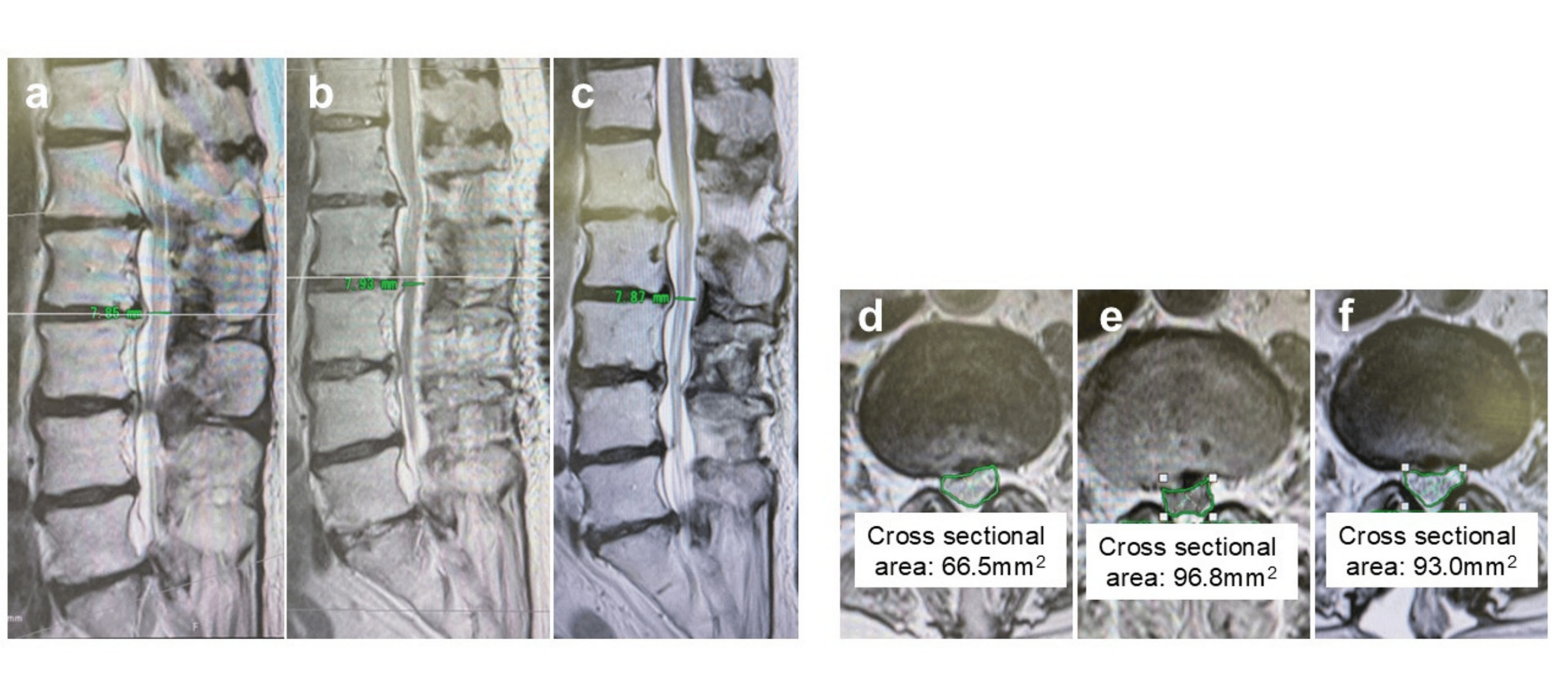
The anteroposterior diameter of the spinal canal and the dural canal cross-sectional area in the L2/3 level on MRI (a–c) The anteroposterior diameter of the spinal canal at the L2/3 level on MRI before surgery, immediately after, and at the three-month follow-up (all were 7.9 mm). (d–f) The cross-sectional area of the spinal canal at L2/3 on MRI before surgery, immediately after, and at the three-month follow-up (66.5 mm^2^ vs. 96.8 mm^2^ vs. 93.0 mm^2^, respectively).
